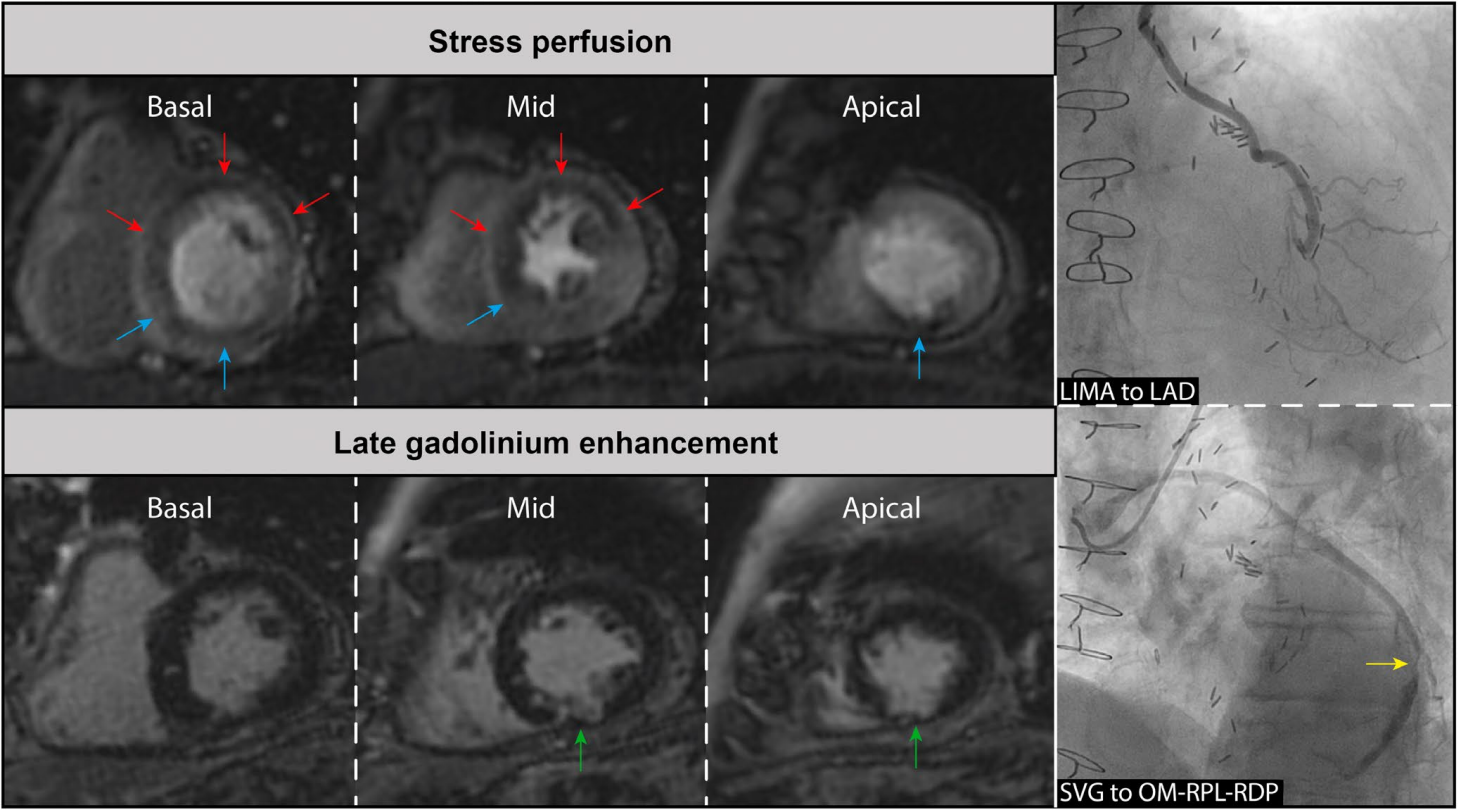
Case example of a false‐positive stress perfusion CMR in a patient with a LIMA graft. Case of a patient with prior CABG (LIMA to LAD and SVG to OM‐RPL‐RDP) who underwent adenosine stress perfusion CMR imaging because of recurrent angina symptoms. CMR showed perfusion defects in the LAD territory; red arrows, as well as the RCA territory; blue arrows. Late gadolinium enhancement imaging revealed the presence of subendocardial scar in the inferior wall (green arrows), the extent of which was smaller than the perfusion defect in this area, suggesting the presence of ischemia in the RCA territory. ICA revealed a patent LIMA to LAD and native LAD distal to the anastomosis, with retrograde filling to two diagonal branches. The SVG to OM‐RPL‐RDP had an obstructive stenosis located between the anastomosis with OM and RPL (yellow arrow). The perfusion defects in the RCA territory can be explained by this SVG lesion. However, a patent LIMA to LAD with intact runoff is observed, which does not explain the observed perfusion defect in the anterior wall of the left ventricle. This relative perfusion defect could be caused by a discrepancy between the time point of contrast arrival through the LIMA graft in comparison to native arteries and the SVG. CABG, coronary artery bypass grafting; CMR, cardiac magnetic resonance imaging; ICA, invasive coronary angiography; LAD, left anterior descending artery; LIMA, left internal mammary artery; OM, obtuse marginal artery; RCA, right coronary artery; RDP, right descending posterior artery; RPL, right posterolateral artery; SVG, saphenous vein graft.
